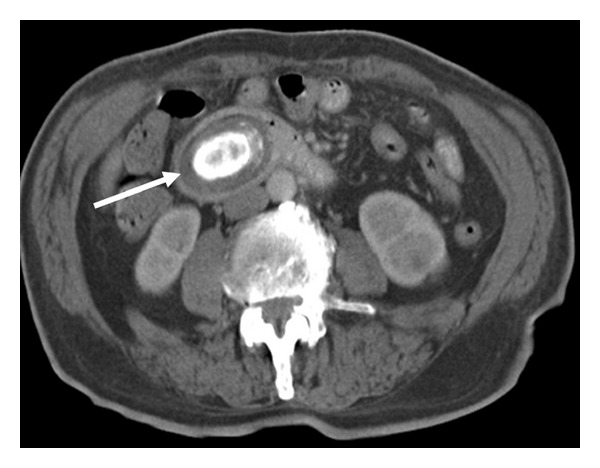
On post-contrast axial CT image a giant gallstone is seen occupying the 3rd and 4th portions of the duodenum.
